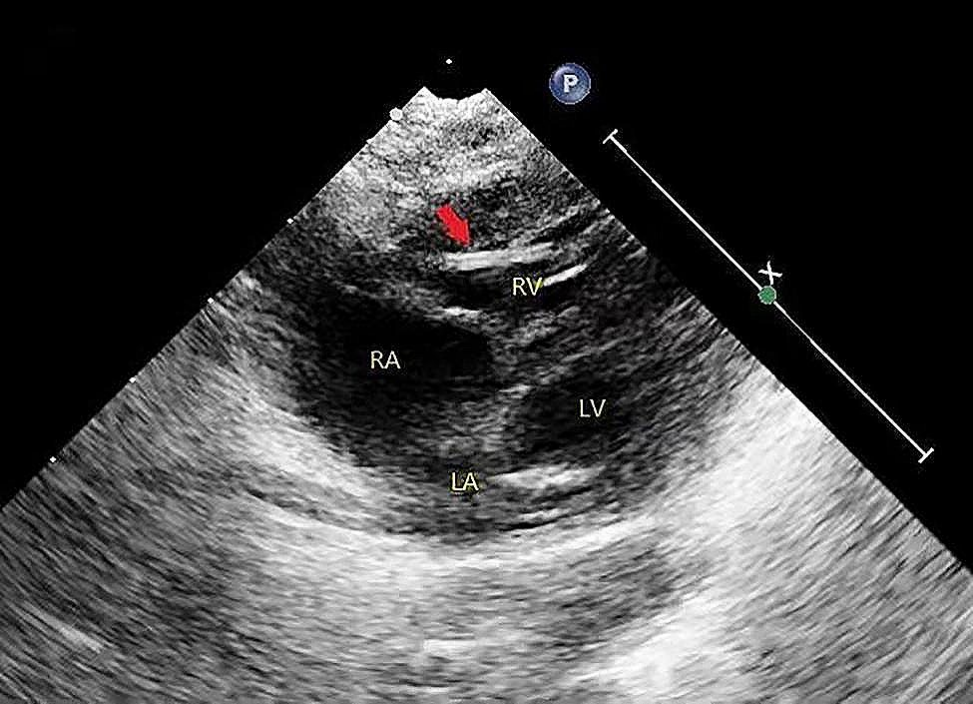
Echocardiography demonstrated a foreign body of 15 mm with line-like strong echo, which was close to the anterior wall of the right ventricle
